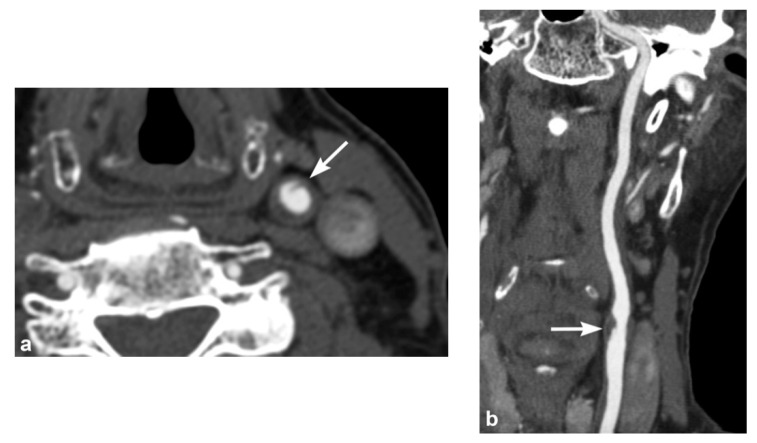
BCVI in a 71-year-old male driver of a motor vehicle accident. (a) Axial multidetector CT angiographic image shows a raised intimal flap in the distal left common carotid artery with focal circumferential wall thickening representing blood dissected into the arterial wall. (b) Coronal multiplanar reformatted CT angiographic images show blood in the arterial wall at the site of injury, leading to focal luminal narrowing without occlusion.
